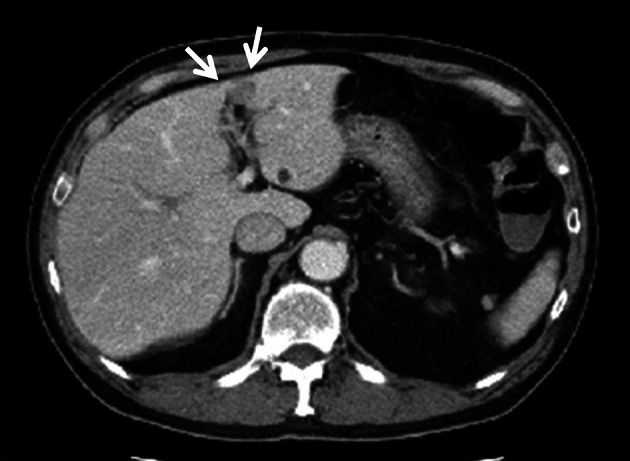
Abdominal CT. The preoperative CT revealed a slightly low density area of 17 mm in diameter was detected in segment 3 of the liver (arrows)
